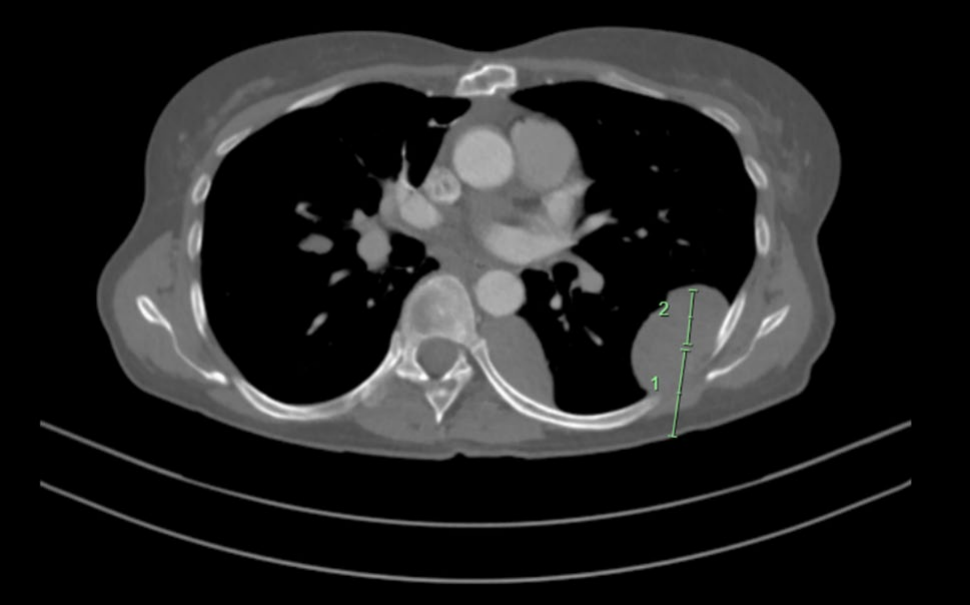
CT-chest with contrast demonstrating the largest subpleural mass in the left lower lobe superior segment measuring 36 mm by 22 mm
